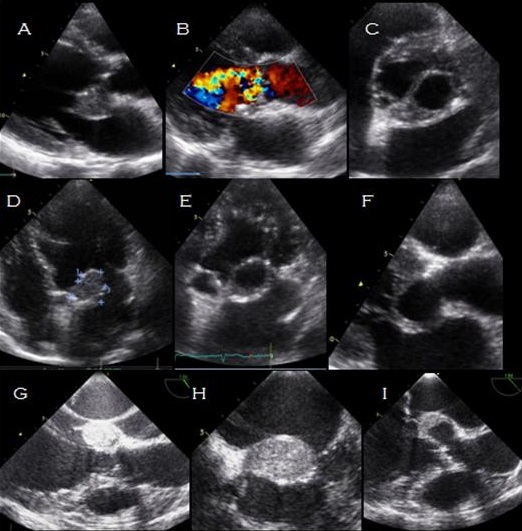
echocardiography images in mycobacterium tuberculosis endocarditis in native valves: A) transthoracic echocardiography, parasternal long-axis view showing a masse located at the anterior mitral valve; B) transthoracic echocardiography, apical 4 chamber view showing the solid part of the mass measuring 23X29mm adherent to the anterior mitral valve; C) transthoracic echocardiography, apical 4 chamber view: showing the empty part of the mass measuring 28X25mm; D) transesophageal echocardiography showing a masse fusing to the mitro-aortic junction; E) transthoracic echocardiography, parasernal short axis view showing a masse fusing in the initial part of the root of the aorta; F) transthoracic echocardiography, parasernal short axis view showing a masse fusing in the the root of the aorta; G) transthoracic echocardiography, parasternal long axis view showing the mass located to the anterior mitral valve and funsig to the mitro aortric junction and along the proximal aortic wall; H) transesophageal echocardiography showing the solid and the empty part of the masse fusing to the mitro-aortic junction, partially drained at left valsalva sinus; I) transthoracic echocardiography, parasternal long axis view moderate aortic insufficiency
